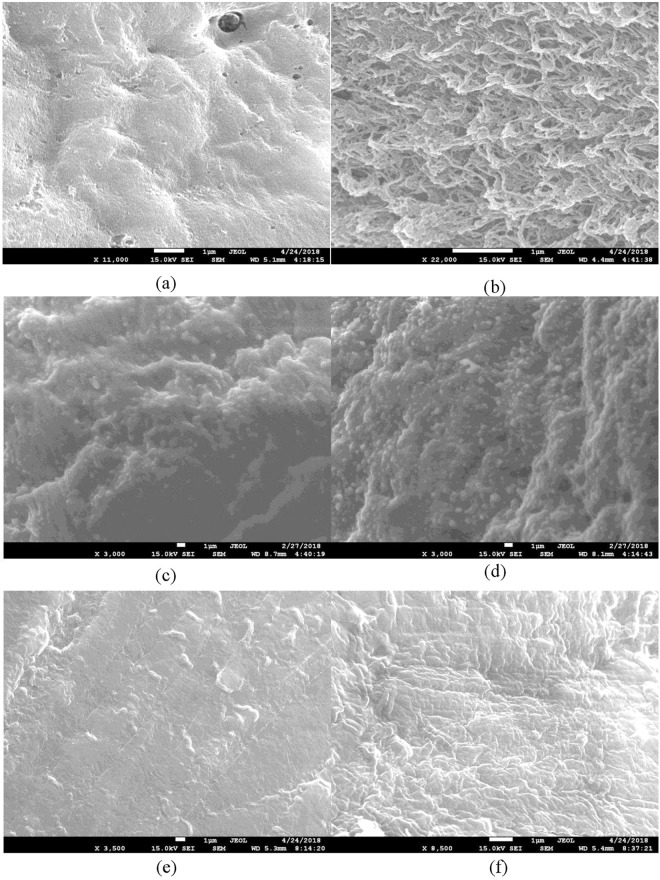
The SEM of (a) Muscle chicken soak with DI water (b) Muscle chicken soak with PAW–ultrasound (c) Rough skin chicken soak with DI water (d) Rough skin chicken soak with PAW–ultrasound (e) Smooth skin chicken soak with DI water (f) Smooth skin chicken soak with PAW–ultrasound.
